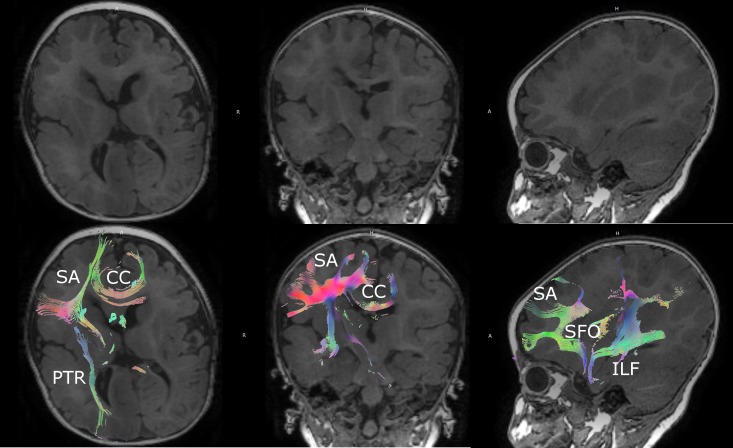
Preoperative magnetic resonance imaging with diffusion tensor imaging Preoperative imaging demonstrating right hemimegencephaly and manual seeding of right hemispheric white matter tracts for preoperative planning and intraoperative neuronavigation. CC: corpus callosum; PTR: posterior thalamic radiations; SA: short association fibers; SFO: superior fronto-occipital fasciculus; ILF: inferior longitudinal fasciculus
